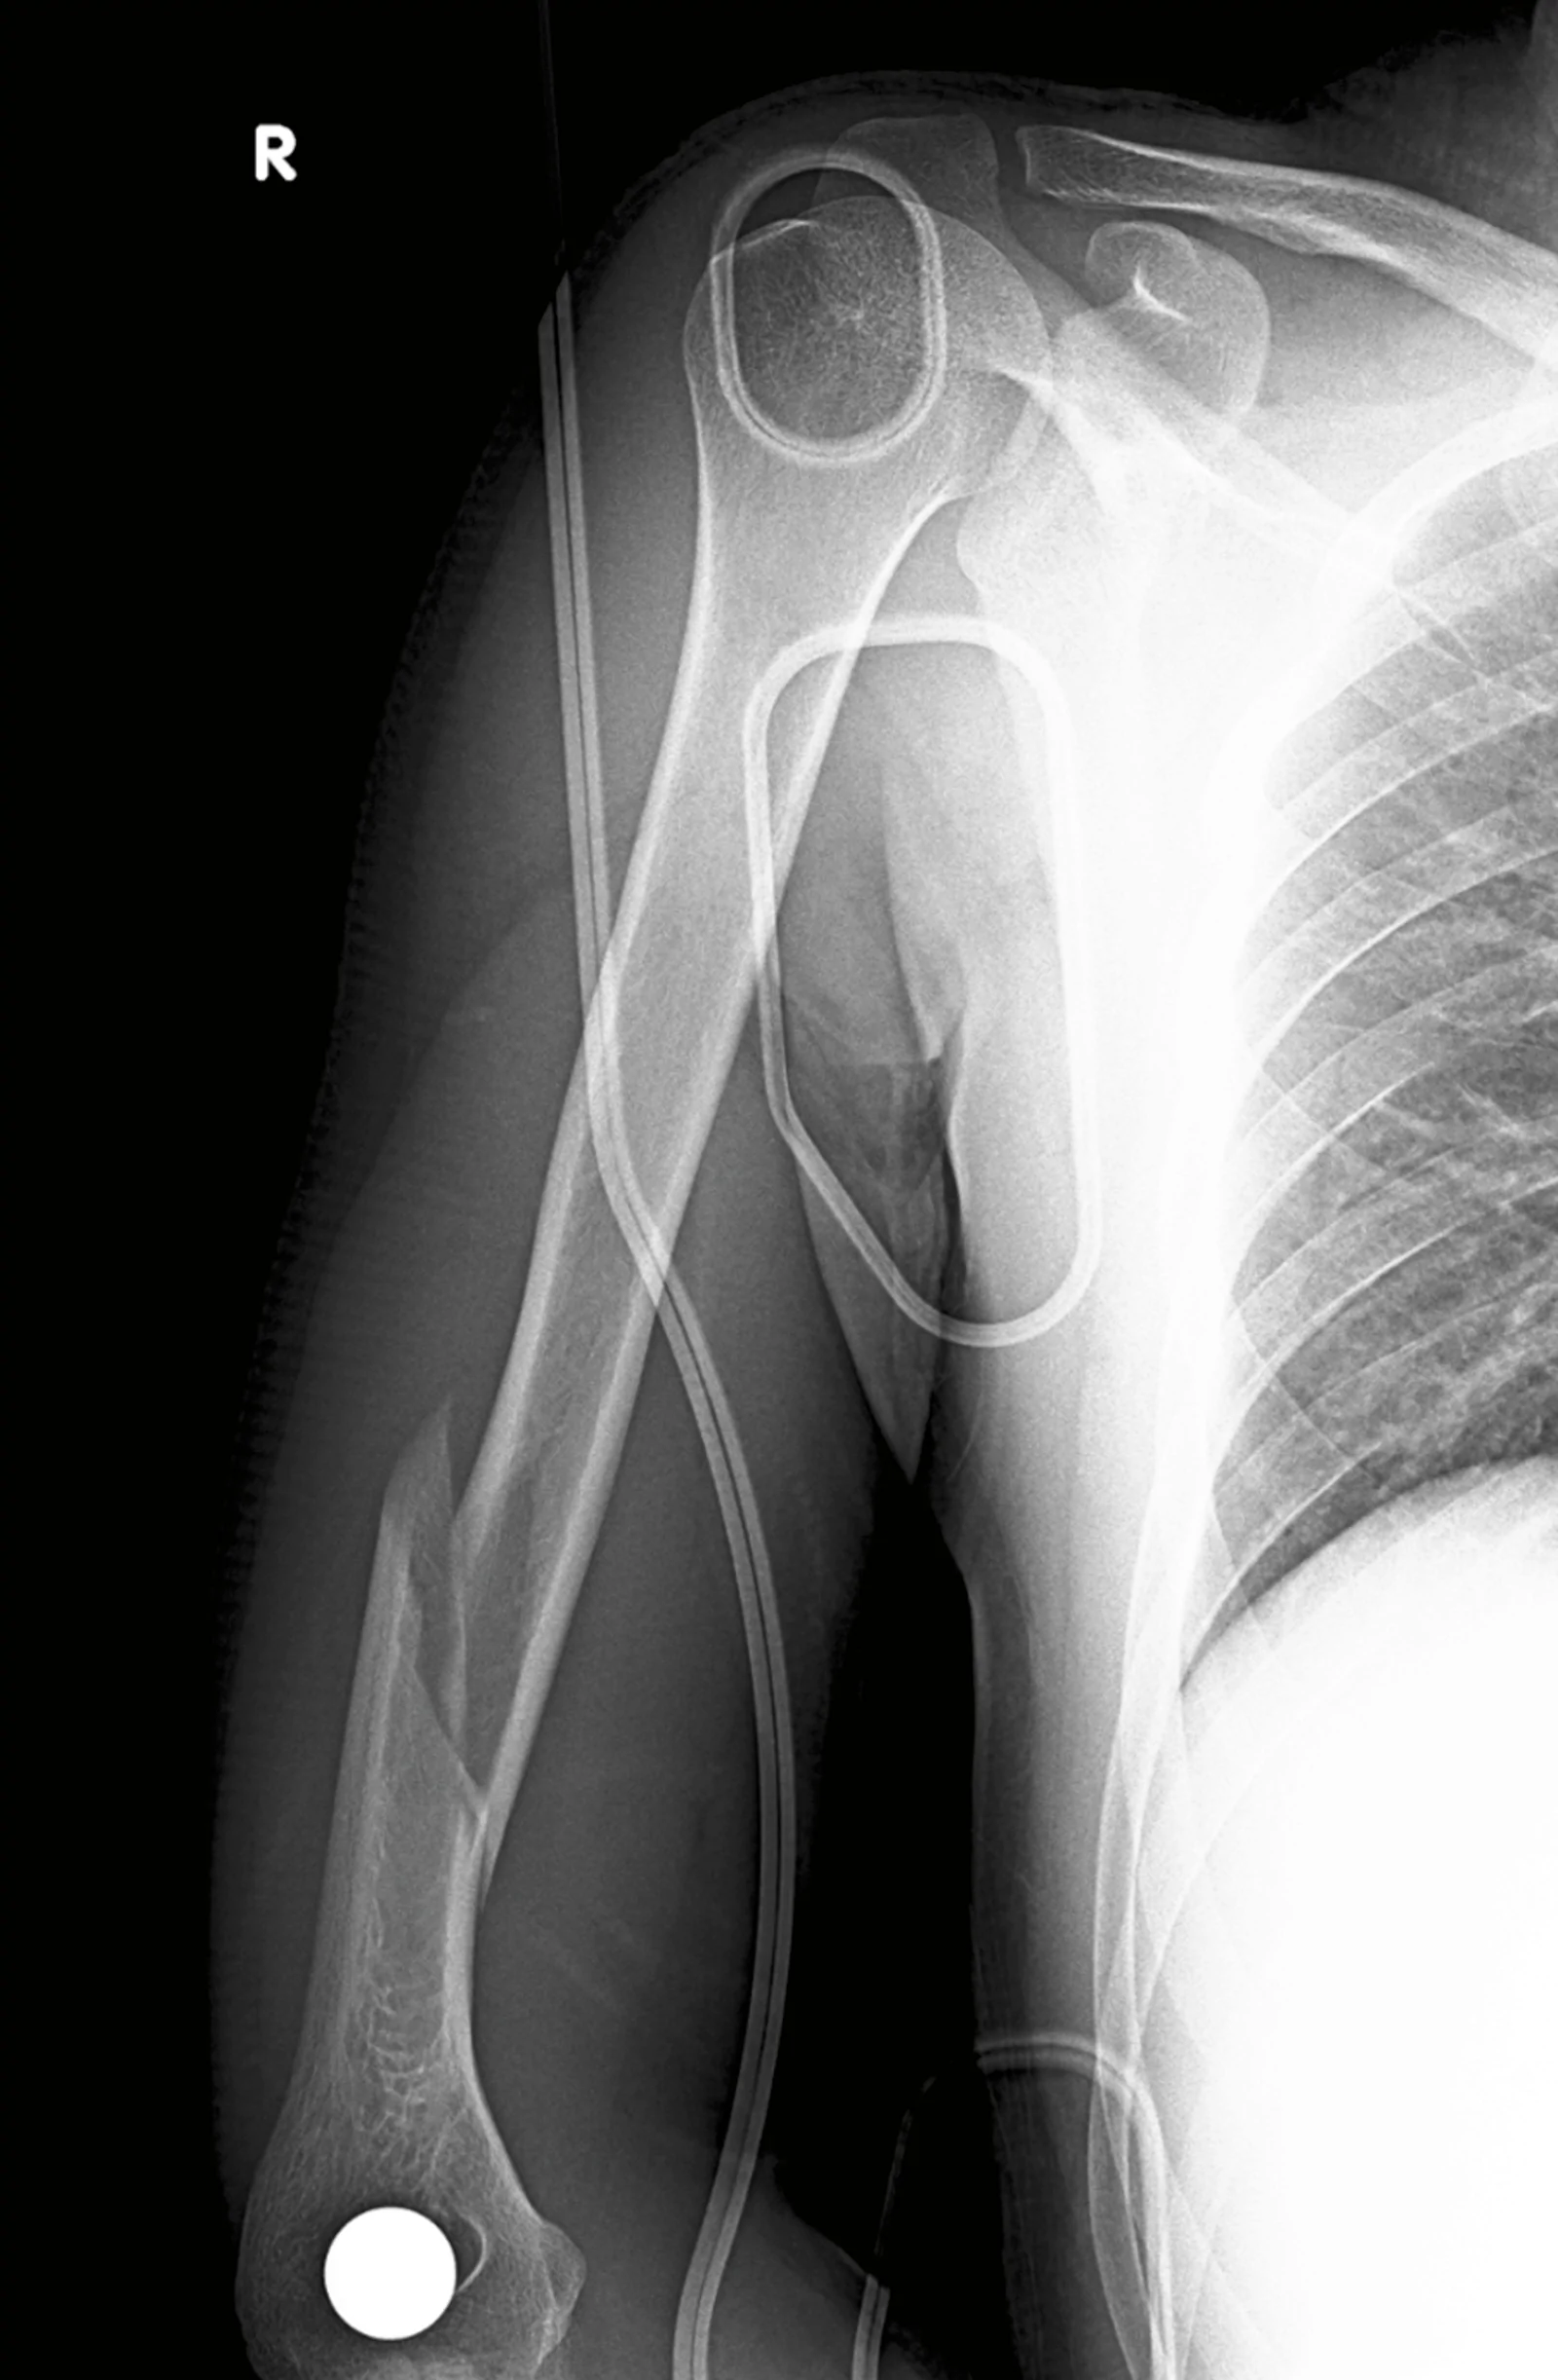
Preoperative radiograph of the humeral shaft fracture. Preoperative anteroposterior radiograph demonstrating an AO/OTA 12A1 spiral fracture of the distal humeral shaft. AO/OTA: Arbeitsgemeinschaft für Osteosynthesefragen/Orthopaedic Trauma Association.
